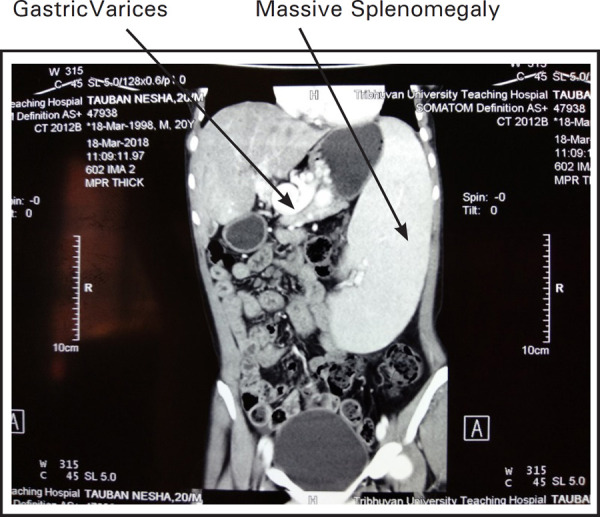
CT scan showing massive splenomegaly with cavernoma and varices.
